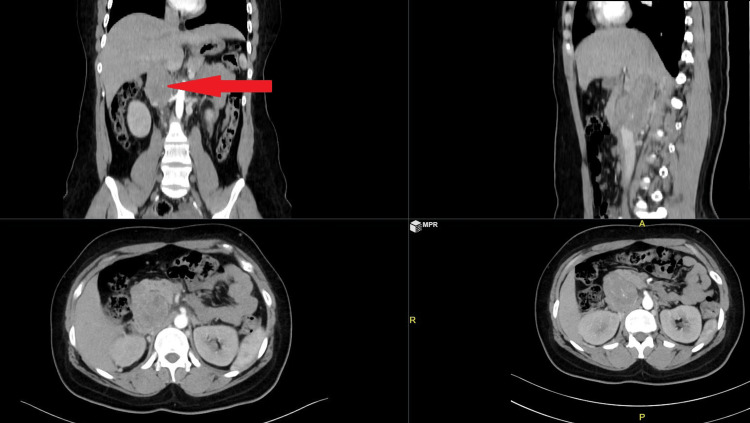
CT abdomen with sub-hepatic mass marked by a red arrow
